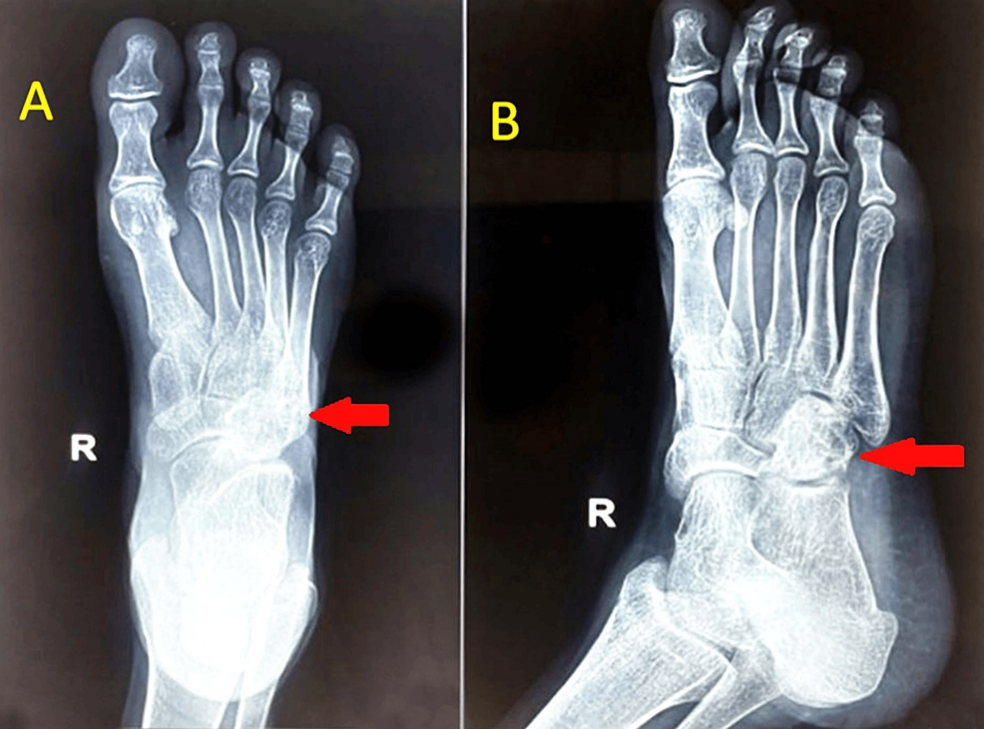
X-ray of the right foot in anteroposterior (A) and oblique (B) views showing an osteolytic lesion over cuboid bones along with soft tissue swelling and diffuse transient osteopenia
